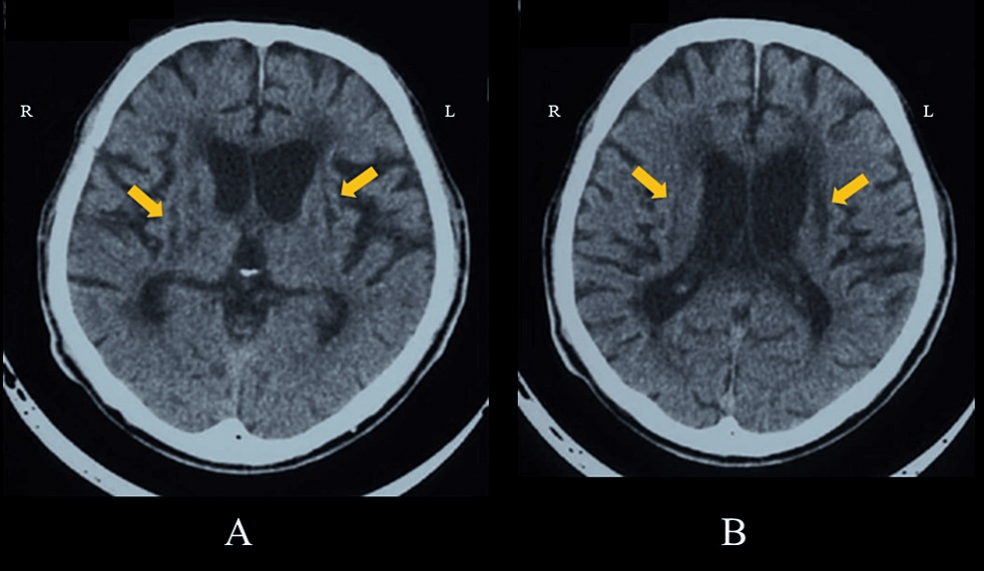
Brain computed tomography (CT) (Case 1). Brain CT revealing low-density areas in the bilateral basal ganglia, internal capsule, and corona radiata (arrow): (A) basal ganglia level slice view and (B) corona radiata level slice view. R: right side, L: left side.
